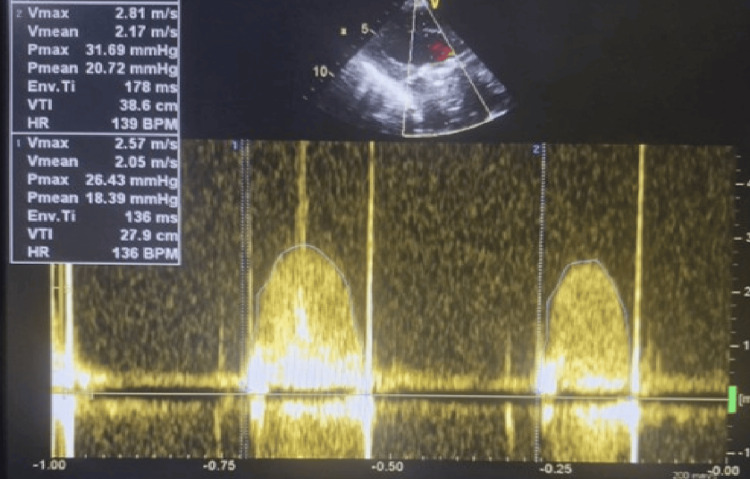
Echocardiography with continuous wave Doppler ultrasound Echocardiography with continuous wave Doppler demonstrating recurrent elevation of transmitral gradients (Pmax1 26.43 mmHg, Pmean1 18.39 mmHg, Pmax2 31.69 mmHg, Pmean2 20.72mmHg), confirming recurrent prosthetic valve obstruction. Pmax - peak gradient; Pmean - mean gradient
